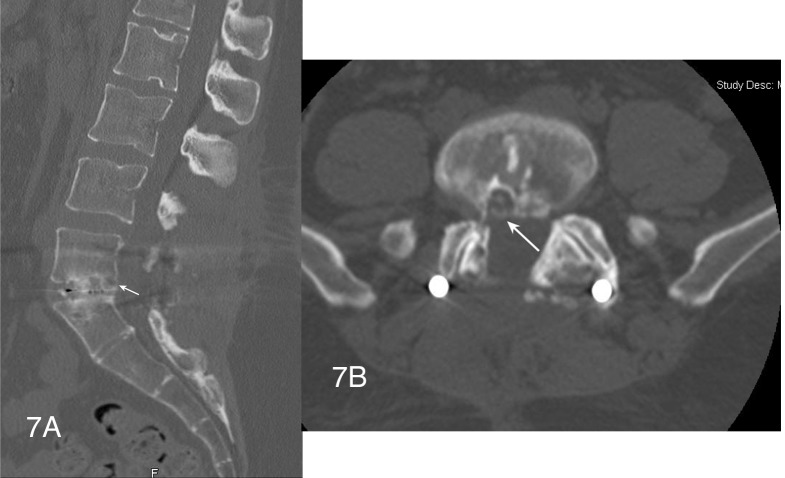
Sagittal and axial postoperative CT images showing calcification along previously noted cyst wall (Figures 7A, 7B) Sagittal and axial postop CT images at 1 year postop displaying a solid interbody fusion. The posterior aspect of where the cyst wall was (arrows) is noted to be calcified.
